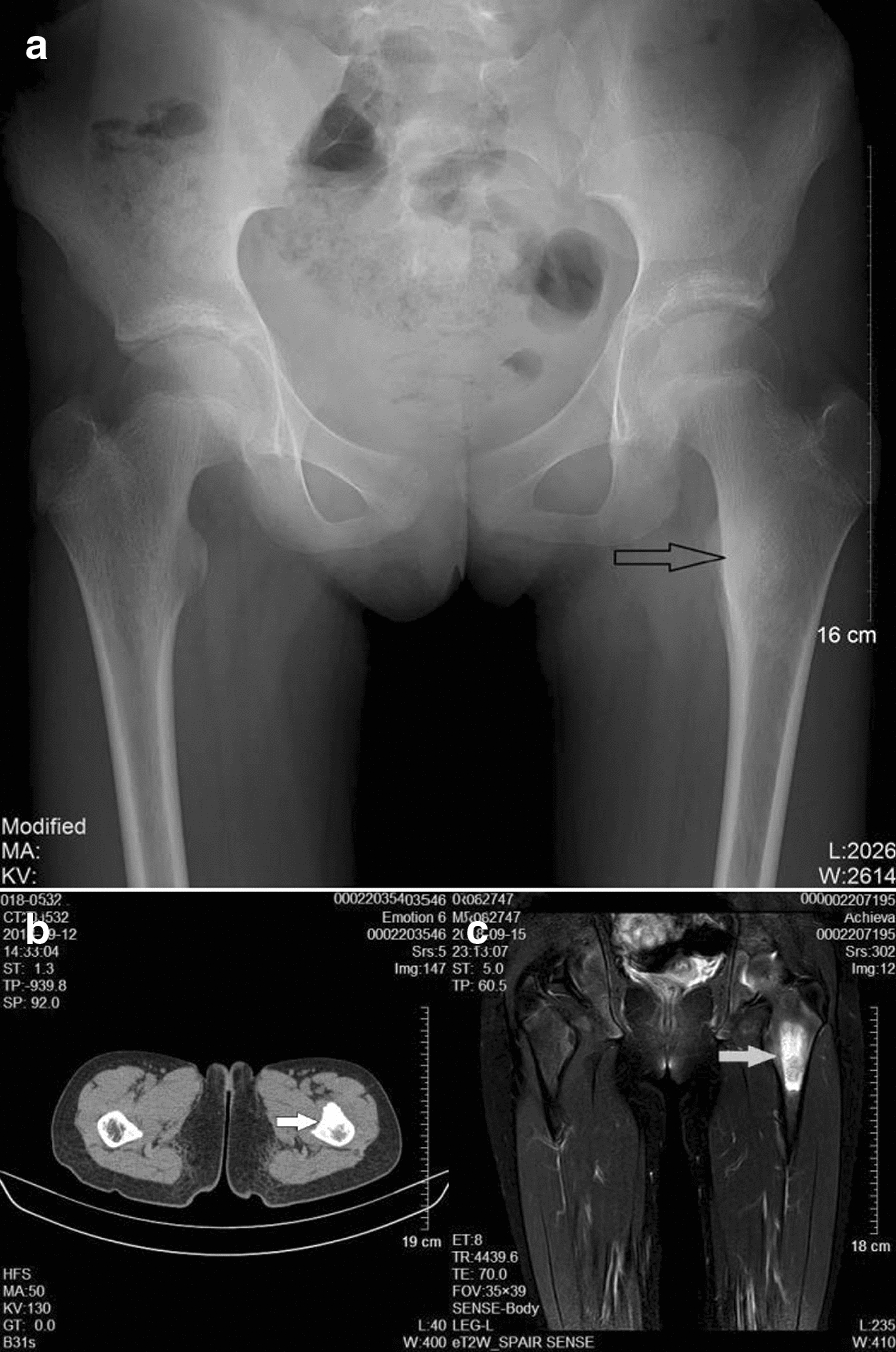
a Preoperative X-ray examinations show increased density (arrow) of the greater trochanter of the left femur in a 15-year-old girl presenting with a 2-month history of left lower leg pain. b, c CT coronal and sagittal image of the lesion (arrow)
